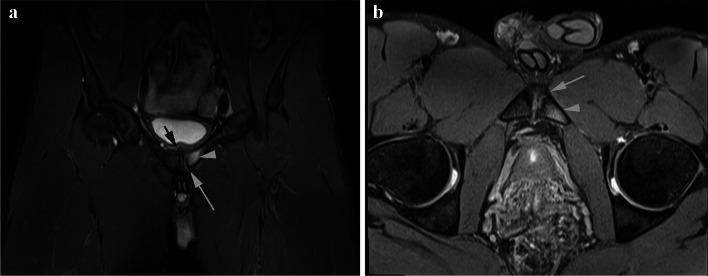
Symptomatic 24-year-old male athlete. a coronal STIR and b axial oblique PD-weighted fat suppressed MR images show left-sided pubic body (arrowhead) and subchondral bone (white arrow) marrow oedema. Right-sided bone marrow and bilateral capsule/aponeurosis soft tissue scored as normal. Symphysis pubis superior bone spurring (black arrow) scored as present
